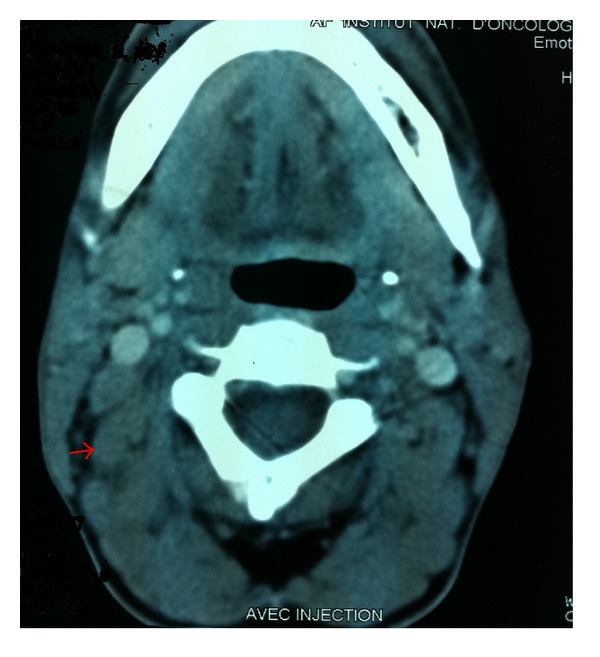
Computed tomography scan of the cervical showed a right cervical lymphadenopathy measuring 3 × 3 cm.
